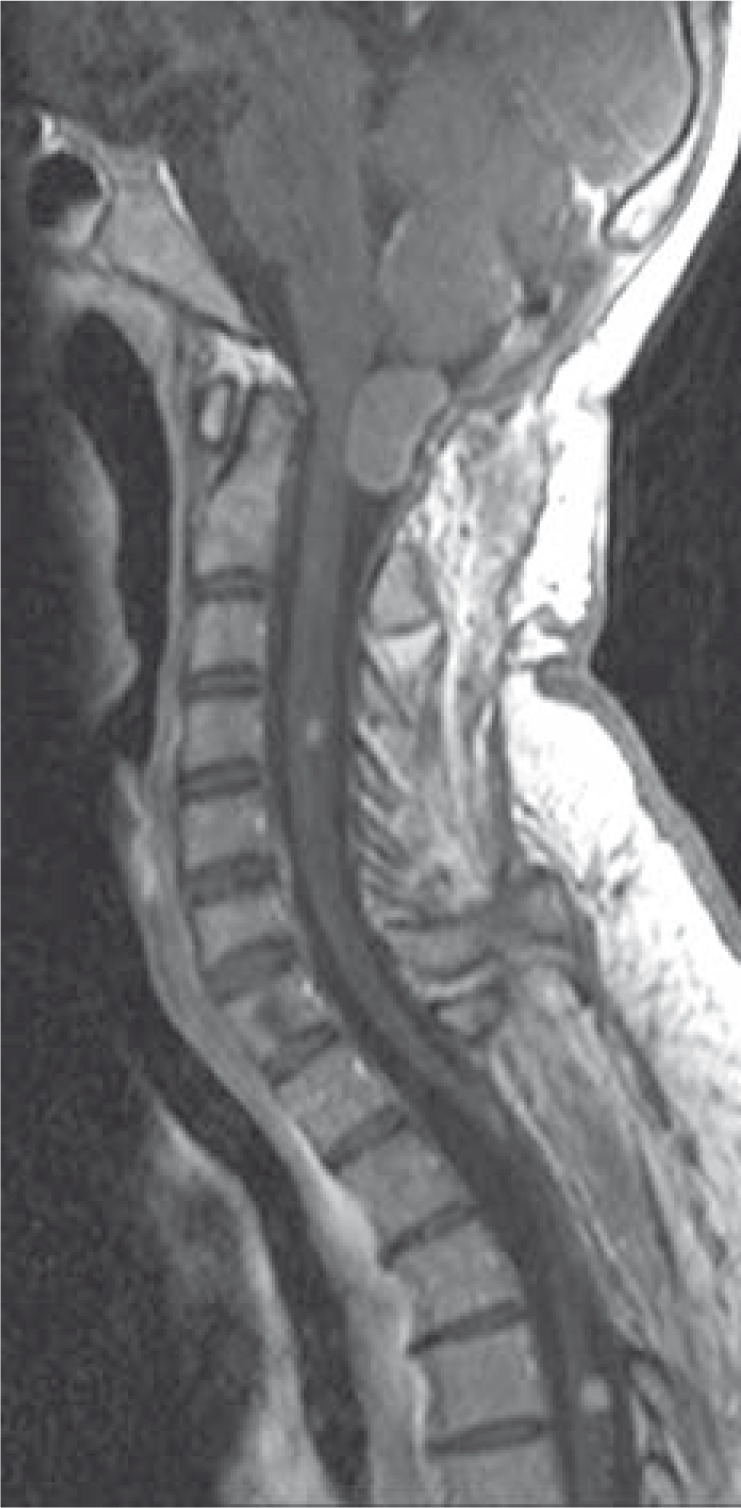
Sagittal T1 post-contrast MR image shows cerebellar haemangioblastoma in the foremen magnum with spinal haemangioblastomas.
